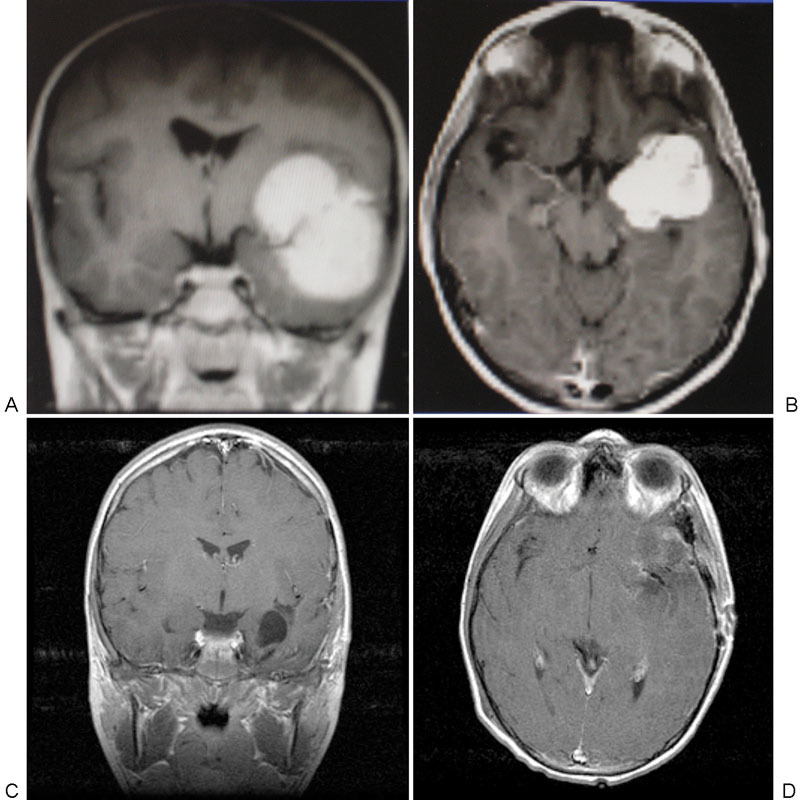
(A) Coronal and (B) axial contrast-enhanced preoperative magnetic resonance imaging (MRI) for patient one showing uniformly enhancing mass measuring 5 × 5 × 4 cm within the left sylvian fissure region. (C) Coronal and (D) axial contrast-enhanced postoperative MRI for patient one showing postoperative changes after microdissection and successful resection of tumor with no significant residual enhancement along resection margins. There has been no delayed recurrence of tumor in this patient at 10 years' follow-up.
